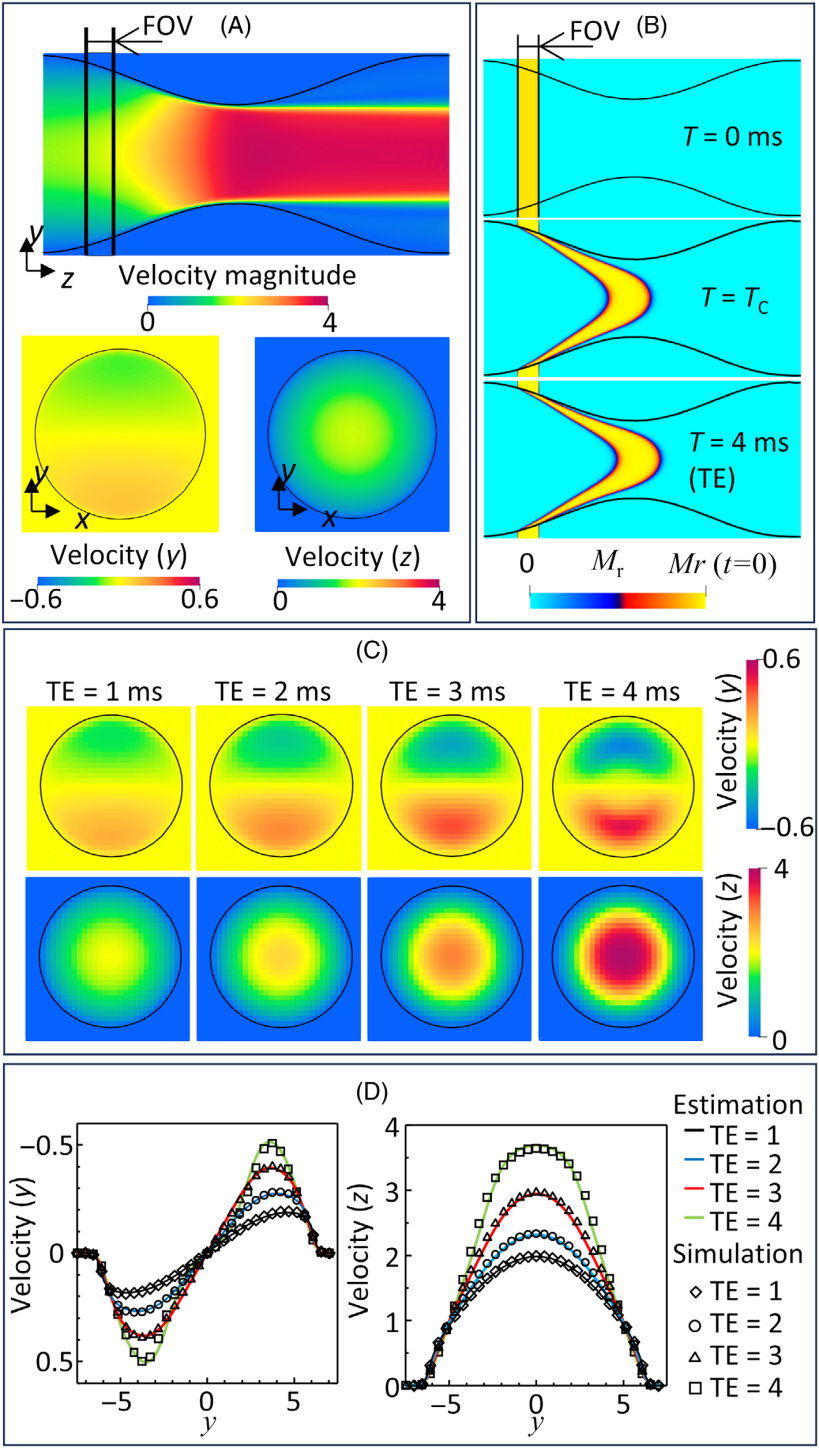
Summary of the displacement artifacts in the MRI‐reconstructed flow velocity fields obtained using Eulerian approach. (A) Input velocity distributions at the center of the field of view (FOV) in the cross‐sectional area of target flow domain (domain A in Figure 3). (B) Representative snapshots of the spatial distribution of the transverse magnetization at t=0, $T_{C}$, and echo time (TE). (C) Reconstructed velocity fields obtained using TE of 1, 2, 3, and 4 ms. (D) Estimated and reconstructed cross‐sectional velocity profiles at the center of the FOV.
